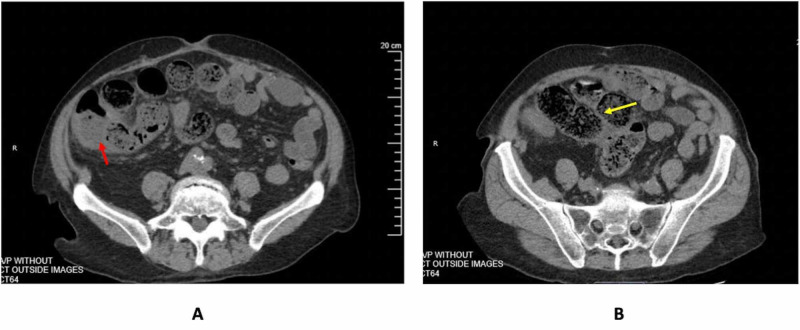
Abdomen/pelvis CT showing right colon thickening and edema (red arrow, A) and small bowel dilation with fecalization (yellow arrow, B).
